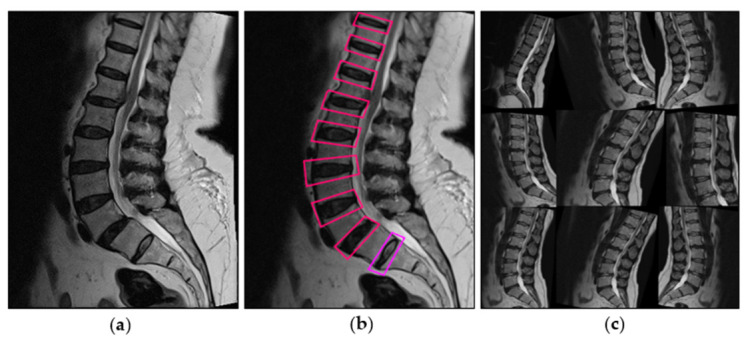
Dataset preparation: sagittal T2-weighted MRI of lumbar spine (a), annotation by a radiologist (b), image generation augmented by horizontal flip, cropping (zoom 0–20%), rotation (between −15° and 15°), shear (±15° horizontal and ±15° vertical) (c).
